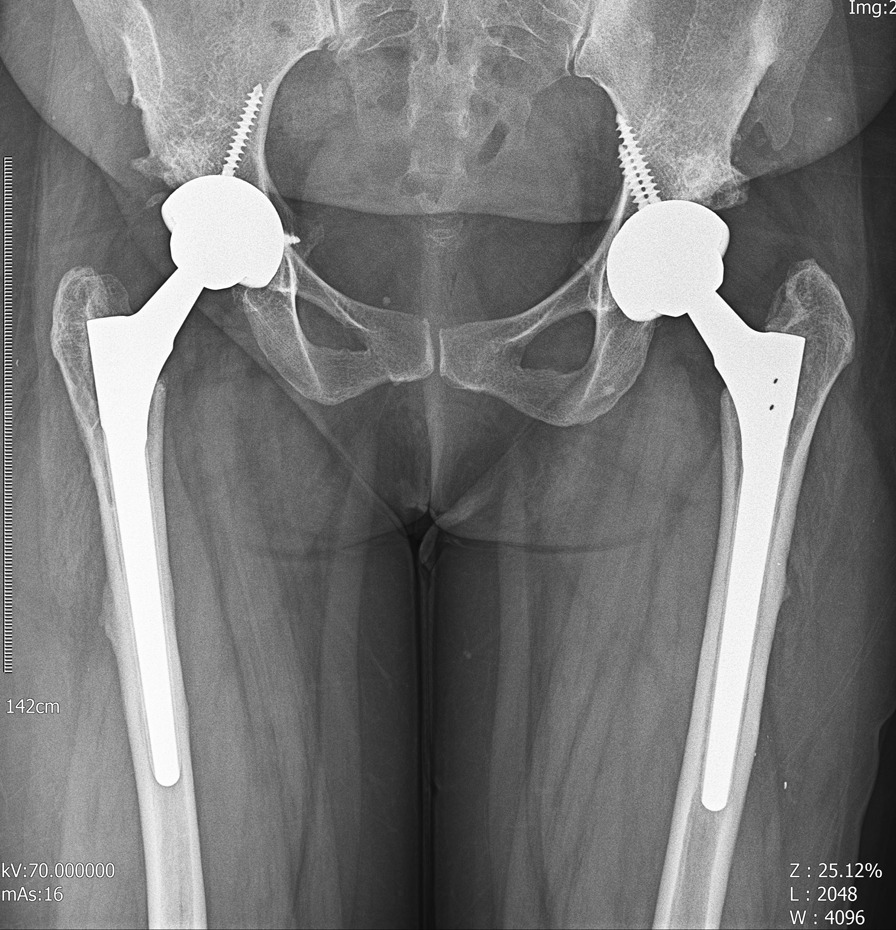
The last pelvic x-ray of our patients 12 months after the surgery
